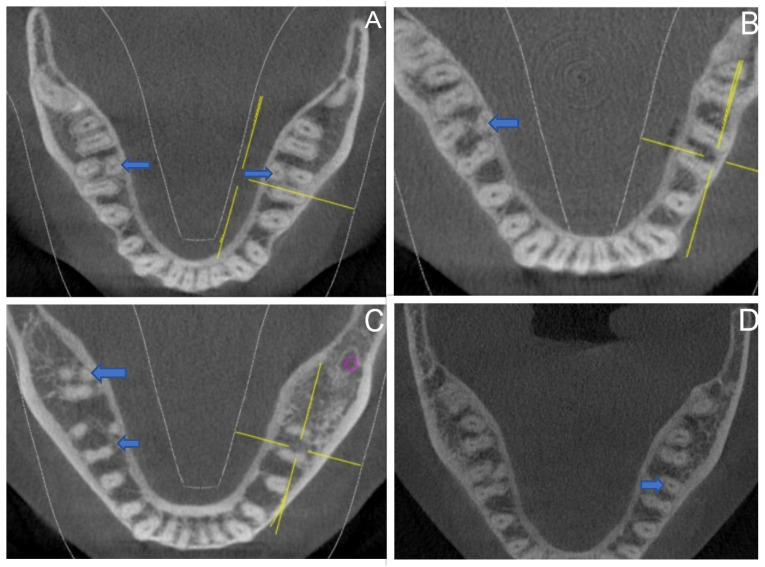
Axial sections of CBCT images indicating (blue arrows) (A) bilateral RE in MFM, (B) unilateral RE in MFM, (C) unilateral RE in MFM and MSM, and (D) an additional distal canal in the main distal root.
